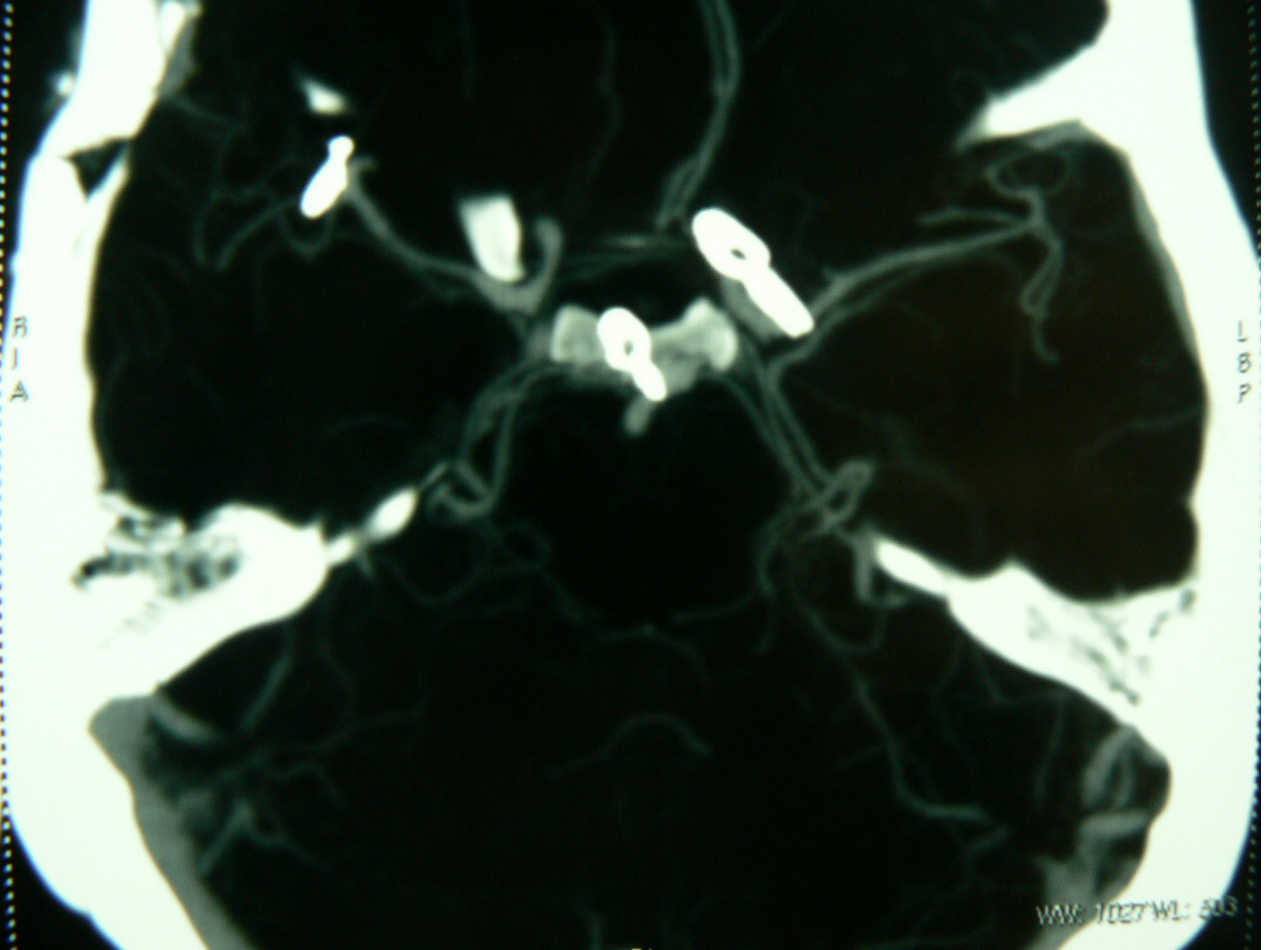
Postoperative CT angiogram of brain showing successful obliteration of all aneurysms.
